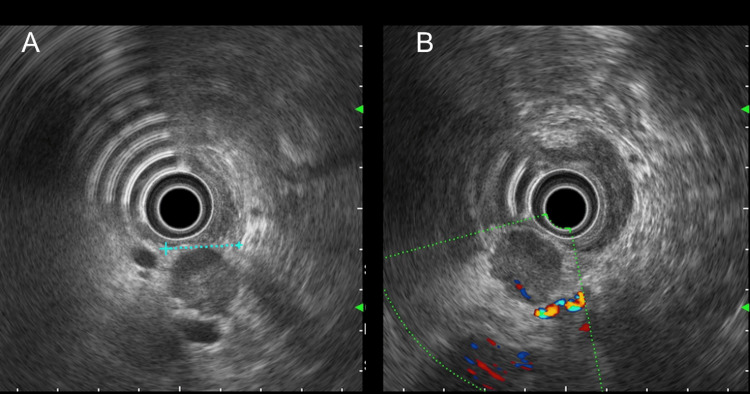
Endoscopic ultrasound images of the pancreatic mass. (A) B-mode image showing a well-defined 18-mm hypoechoic mass in the pancreatic head. (B) Doppler image demonstrating peripheral blood flow within the mass.
